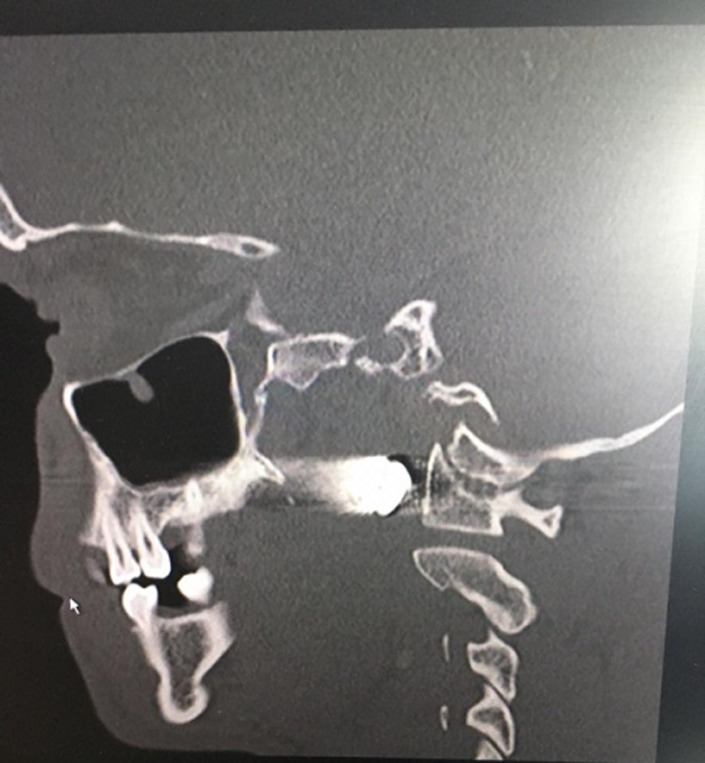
sagittal view of the computed tomography scan
